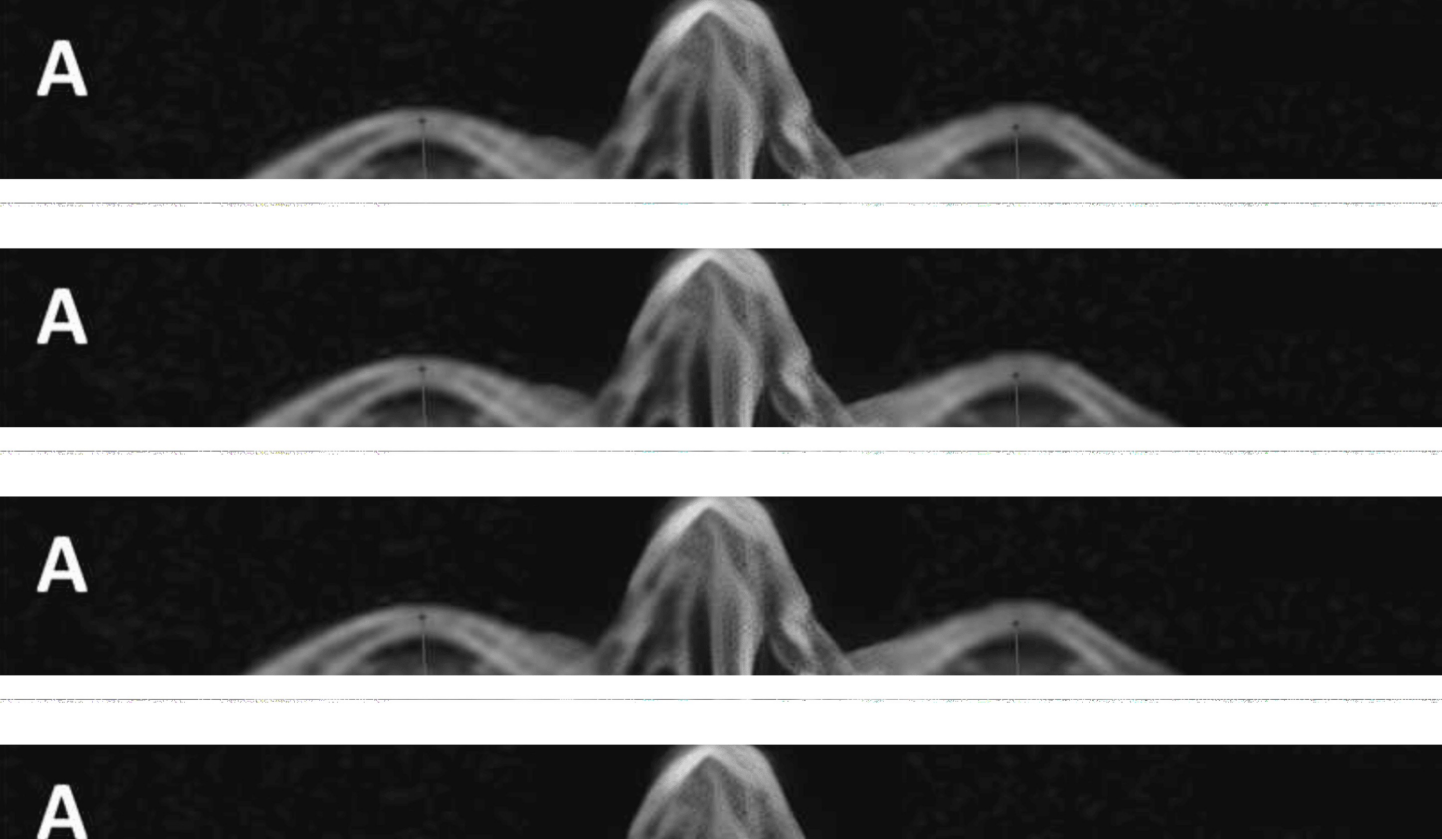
MRI scans showcasing enophthalmos. Enophthalmos, more pronounced on the left side, is evident by measurements from the intercanthal line to the orbital apex (16 mm for the OD and 13.5 mm for the OS) (A). Clinical presentation of the case, photographed from below, to show comparative left enophthalmos (blue line, B), and en-face, to show reduced size of the palpebral aperture to the OS (C). OD: right eye; OS: left eye.
